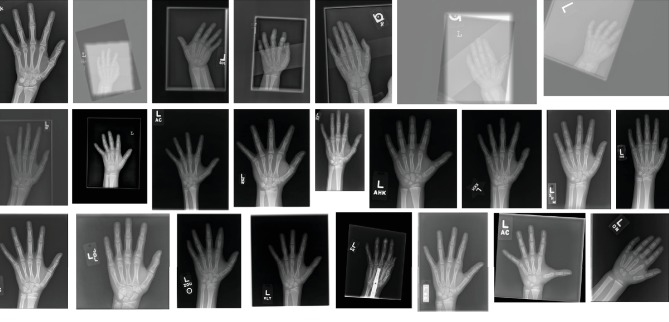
A close-up of part of data in RSNA dataset. Different radiographs vary in size and height-width ratio.
